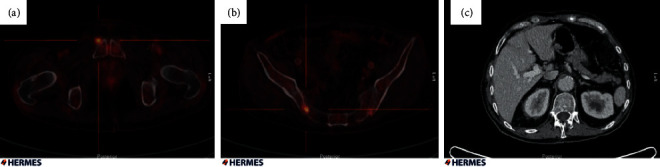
Elevated ^18^F-FDG uptake localized corresponding to (a) the right cheek, cutaneous portion of the back head (occipital) and in one lymph node at the neck and (b) several foci in the pelvic bones, and a soft tissue process ahead of right os pubis showing increased ^18^F-FDG activities. (c) The CT scan showing pathological edematous appearance of the kidney surrounded by adipose tissue.
